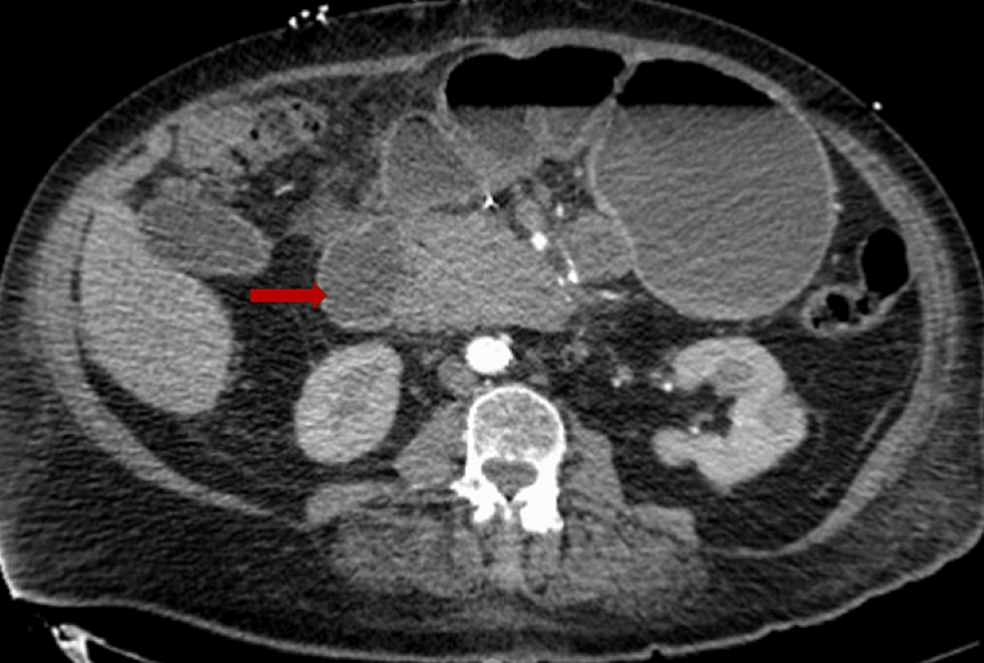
Axial view of computed tomography (CT) of the abdomen demonstrating a lobular soft tissue mass involving the duodenum (red arrow)
